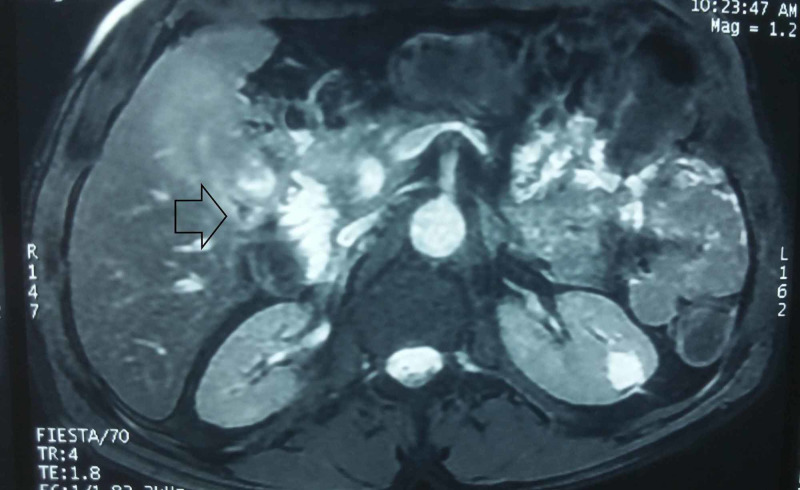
Bili-Magnetic resonance imaging showing suspicious irregular thickening of the gallbladder wall (black arrow).
